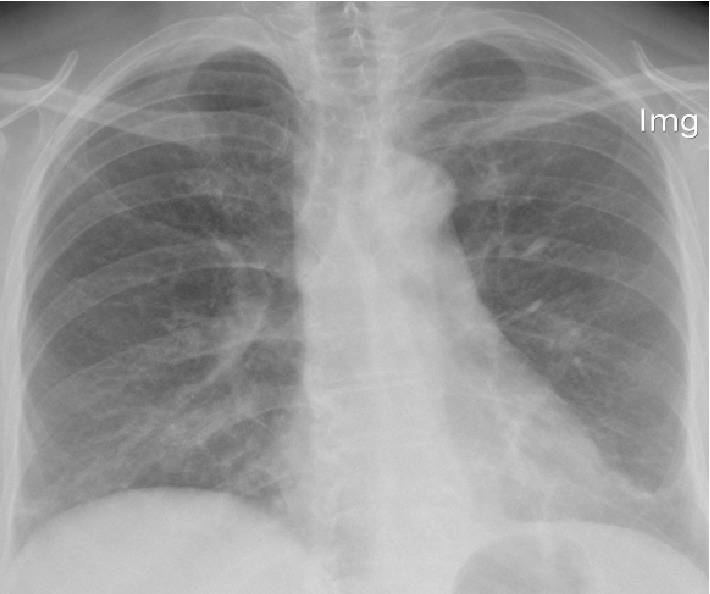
Chest X-ray shows patchy opacifications in right mid zone and reticular nodular shadows in mid zones of both lung fields.
